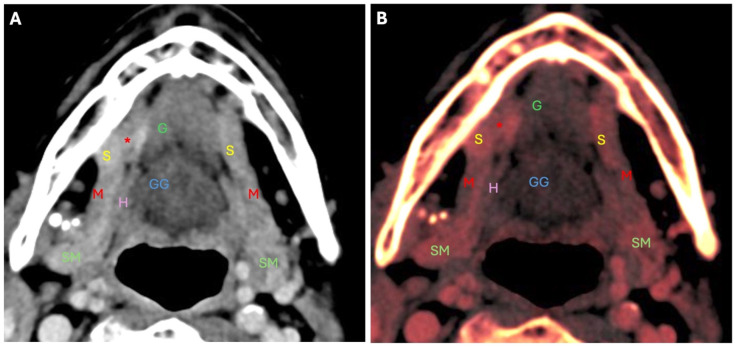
The second patient: dual-energy contrast-enhanced CT neck. A 70-year-old female who presented with nonspecific neck discomfort, lacking palpable masses. Initial imaging (ultrasound and CT) was inconclusive. MRI revealed a right sublingual gland herniation through a mylohyoid defect (mylohyoid boutonniere). In fact, this stable lesion had been present, unrecognized, for three years based on prior imaging. The axial CT image (A) shows a moderately enhancing nodular structure (S) in the right submandibular space, with a narrowed neck extending through a defect of the mylohyoid muscle (*) into the sublingual space. Note a partially imaged venous malformation with phleboliths in the right submandibular space, an incidental finding. An iodine map (B) discovered that the nodular structure has same degree of enhancement as the contralateral sublingual gland and both submandibular glands. S: sublingual gland; SM: submandibular gland; M: mylohyoid muscle; *: boutonniere; G: geniohyoid muscle; GG: genioglossus muscle; H: hyoglossus muscle.
